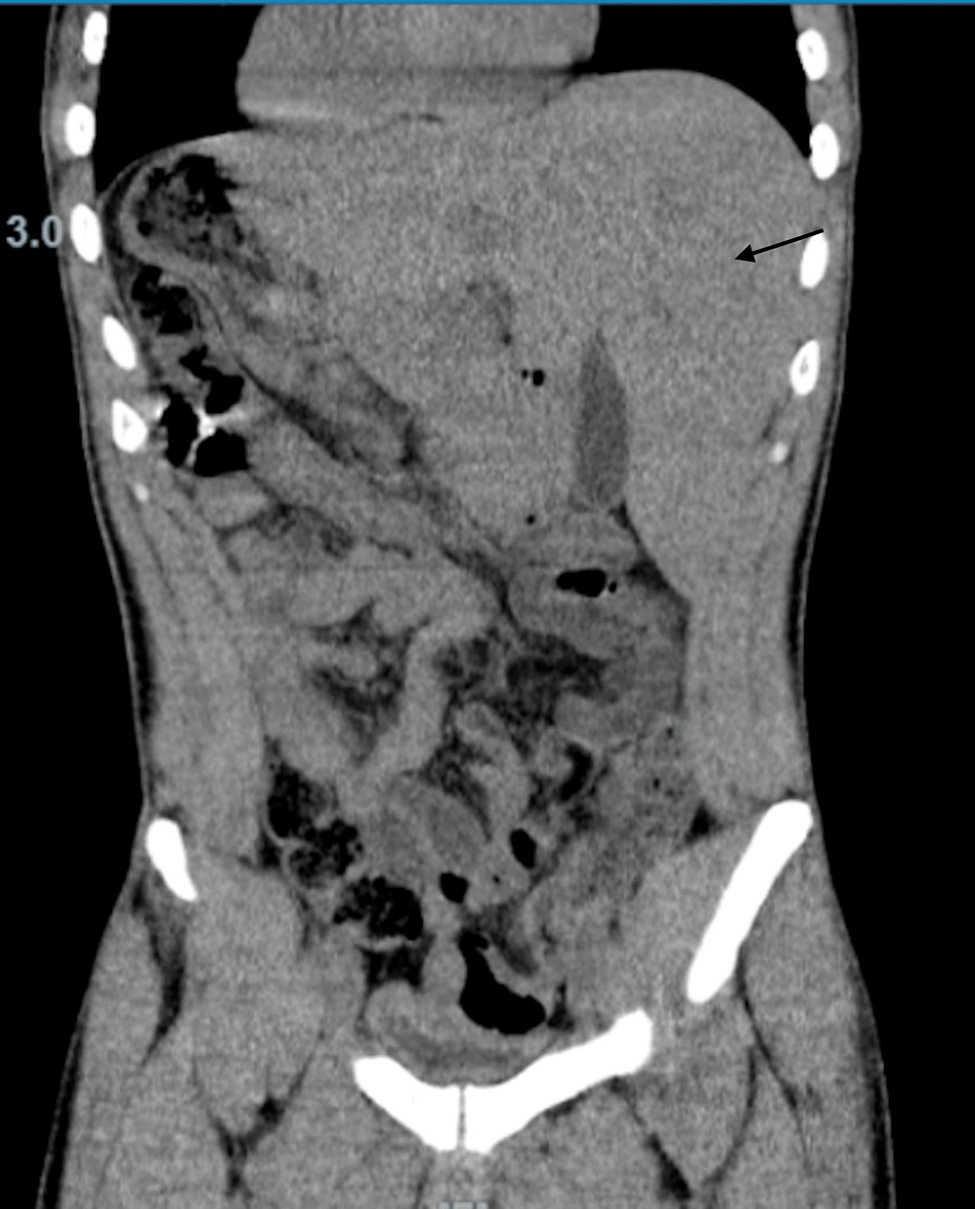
CT abdomen and pelvis w/o contrast (coronal view): situs inversus and dextrocardia. No acute abnormality in the abdomen or pelvis; bronchitis and bronchiolitis; left-sided liver (situs inversus), and the gallbladder is not distended.
